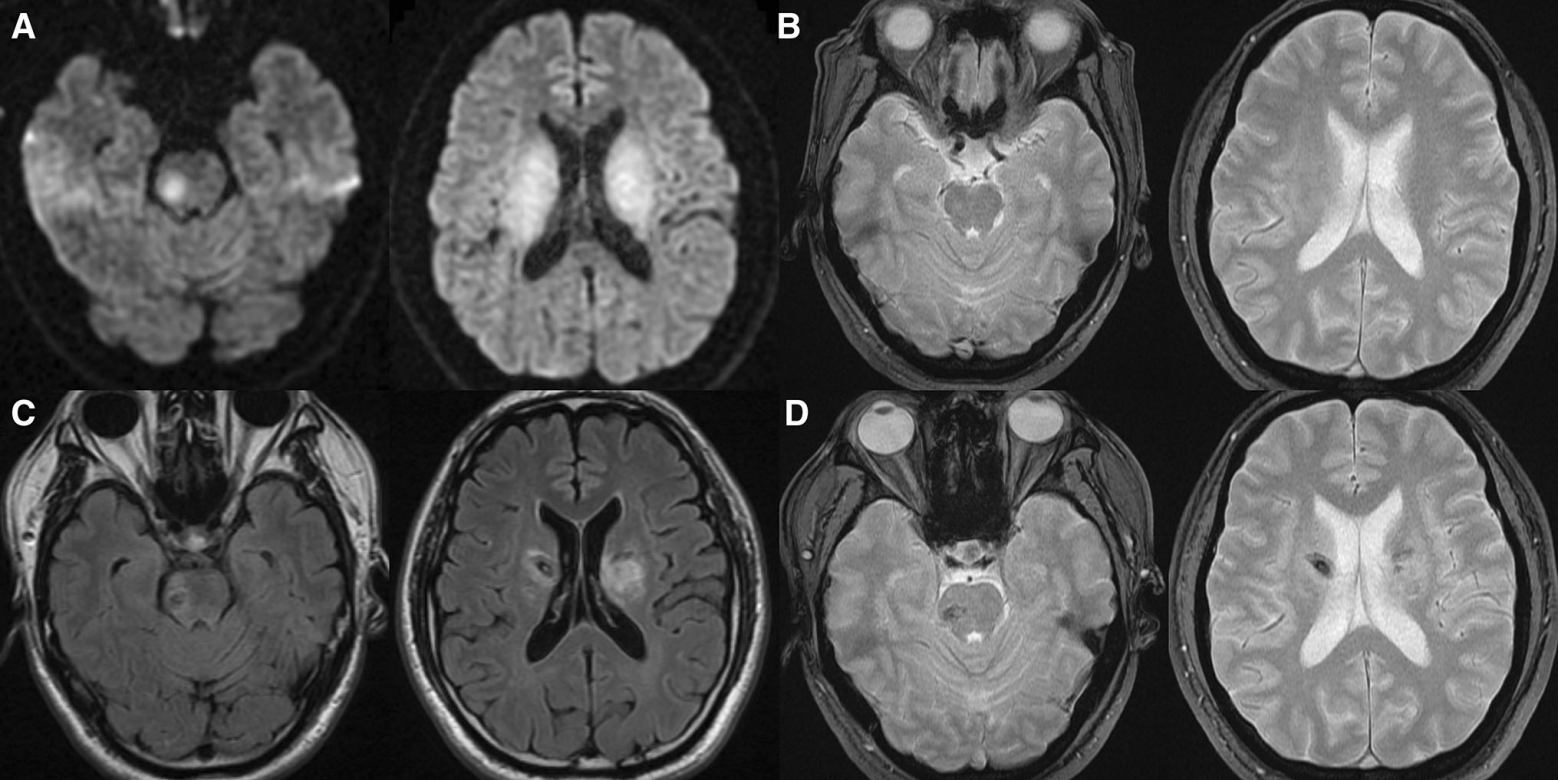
Initial and follow-up magnetic resonance images (MRI) at the second event. The initial MRI (a, b) shows high signal intensities in right pons and both centrum semiovale in diffusion-weighted image (DWI, a), without hemorrhagic lesion in gradient-echo (GRE, b). The follow-up MRI (c, d) shows decreased extent of edema on fluid-attenuated inversion recovery (FLAIR) images (c) and interval development of multiple hemorrhages on GRE (d) in the affected areas
